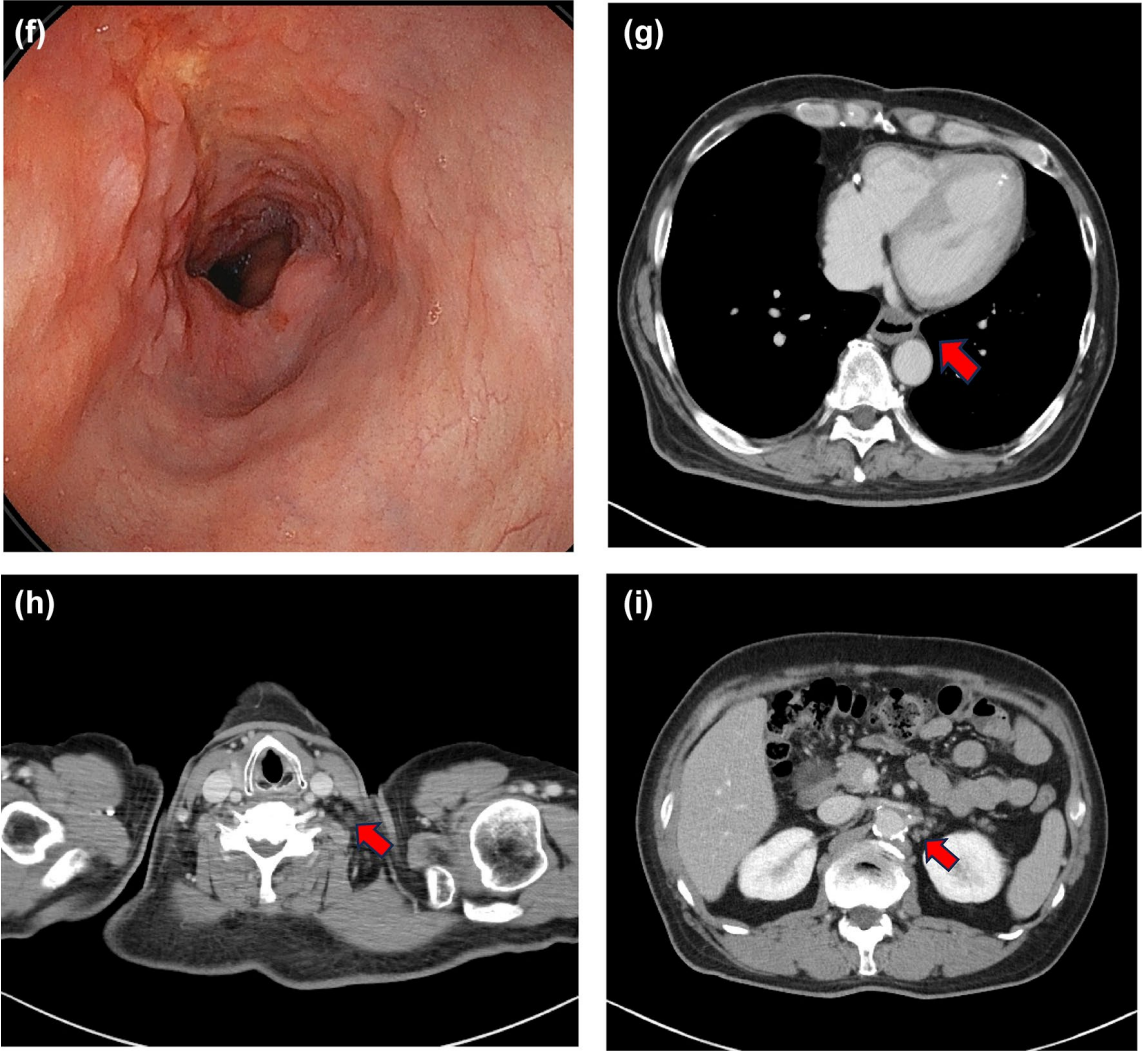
Imaging for Case 2: Initial EGD showing a Type 2 lesion around the EGJ with 8 cm esophageal invasion (a). CT showing enlarged #112-aoA LNs invading the thoracic aorta (b), #104L (c), and #16 LNs (d). Treatment course (e). EGD showing marked shrinkage of the primary tumor (f) and CT showing release of the aortic invasion due to #112aoA LN shrinkage (i) and #104L LN (g) and #16 LN (h) shrinkage after the induction of ICI-combined chemotherapy. EGD, esophagogastroduodenoscopy; CT, computed tomography; LN, lymph node; ICI, immune checkpoint inhibitor
